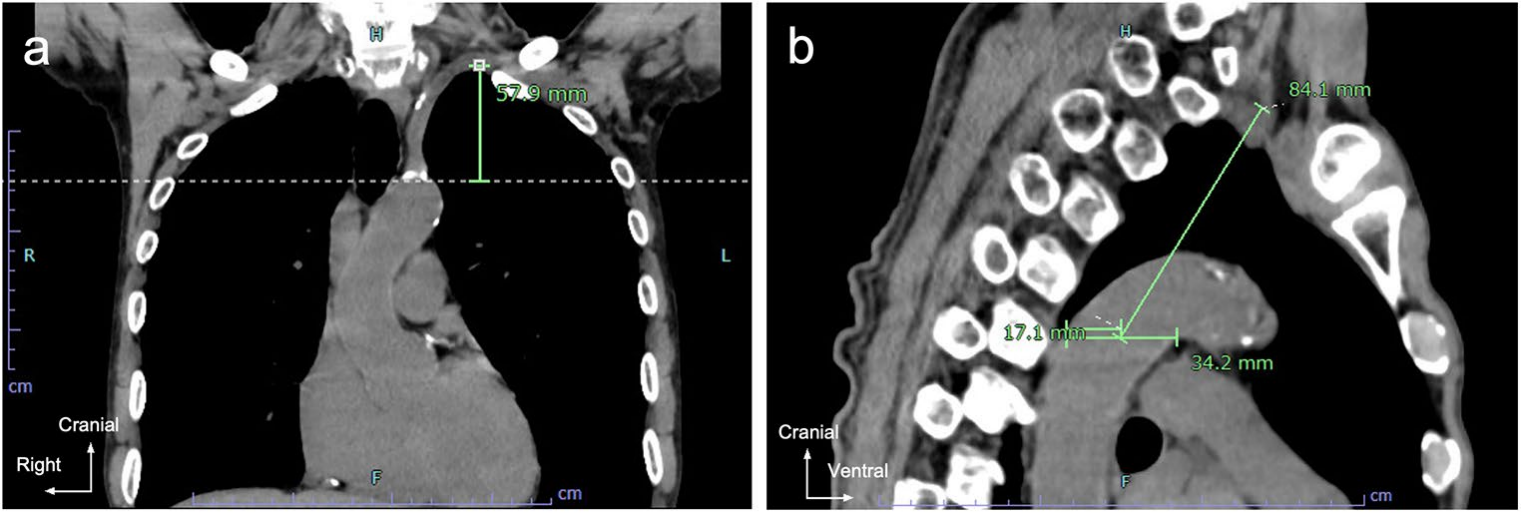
Chest computed tomography images during inspiration. The green line segments indicate the measured distance between the landmarks and correspond to the double arrows in Fig. 1. a Coronal plane. b Sagittal plane
